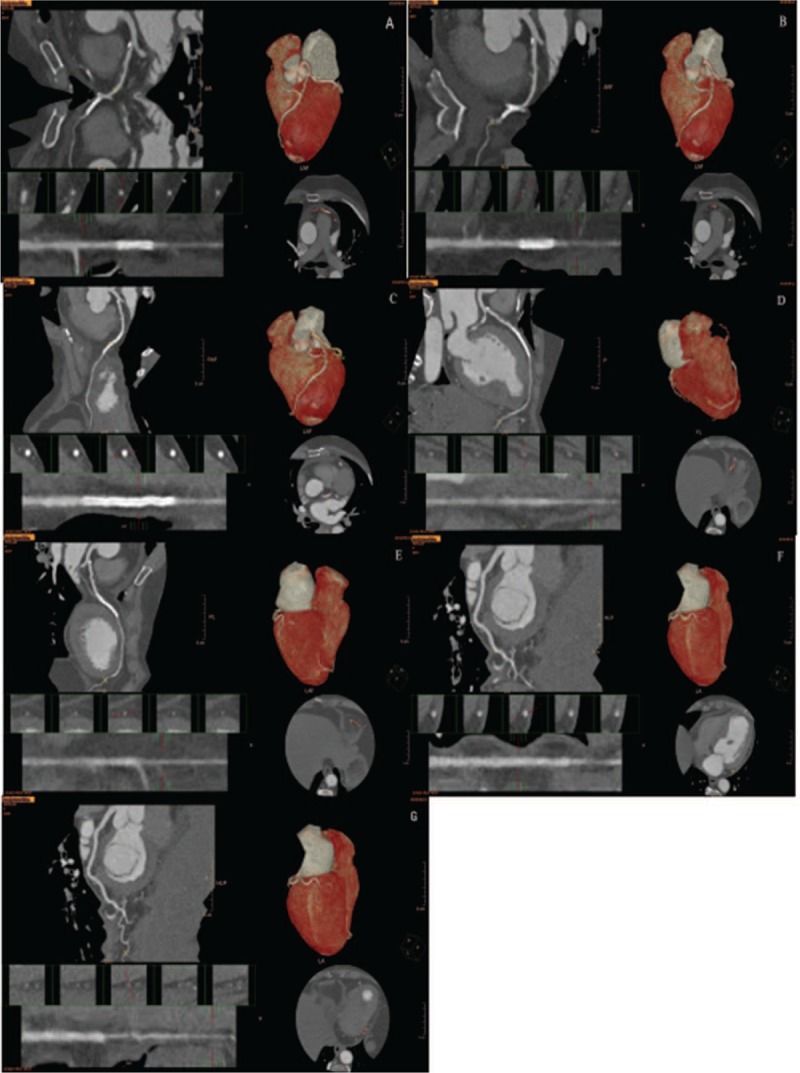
Computed tomography angiography showed that the RCA was not visible from its origin at the aortic root. The RCA originated from the LAD artery. The RCA was short and small with a rough wall. The coronary artery stent could be seen locally, and the corresponding lumen was unobstructed. LAD = left anterior descending, RCA = right coronary artery.
